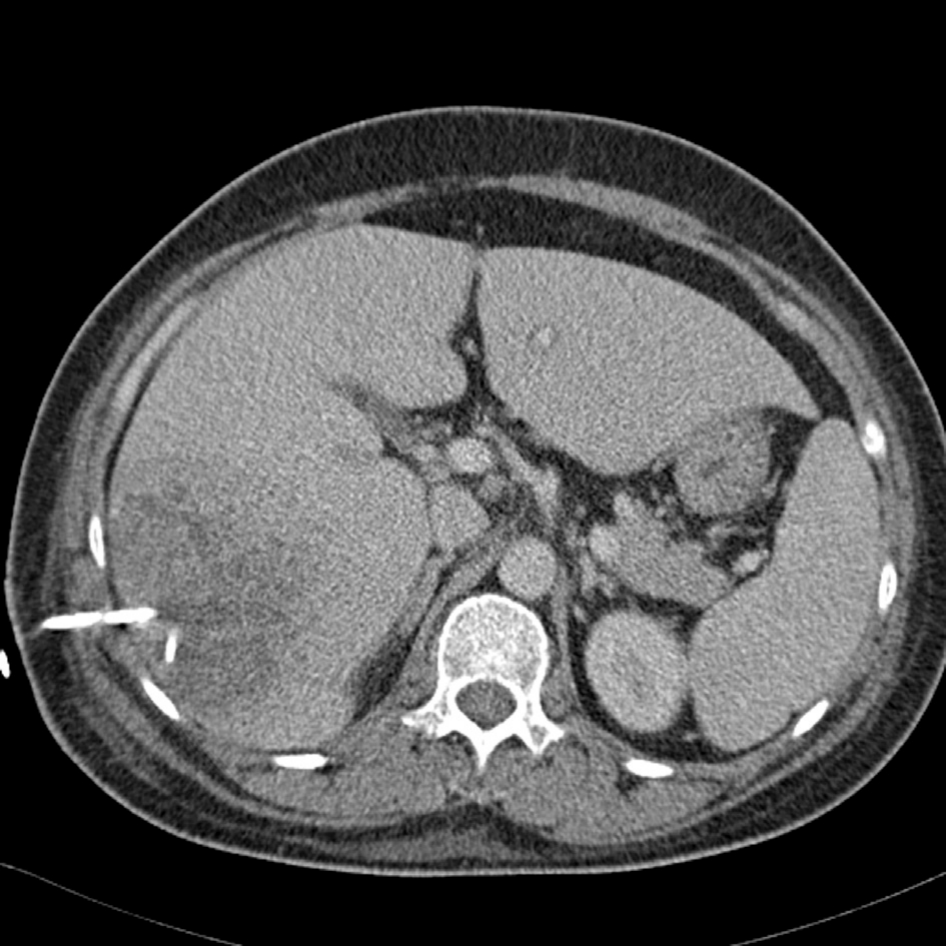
Abdominal CT scan showed a 7.3 × 7.5 cm inhomogeneous abscess in liver segment 6. A therapeutic pigtail catheter was inserted.
